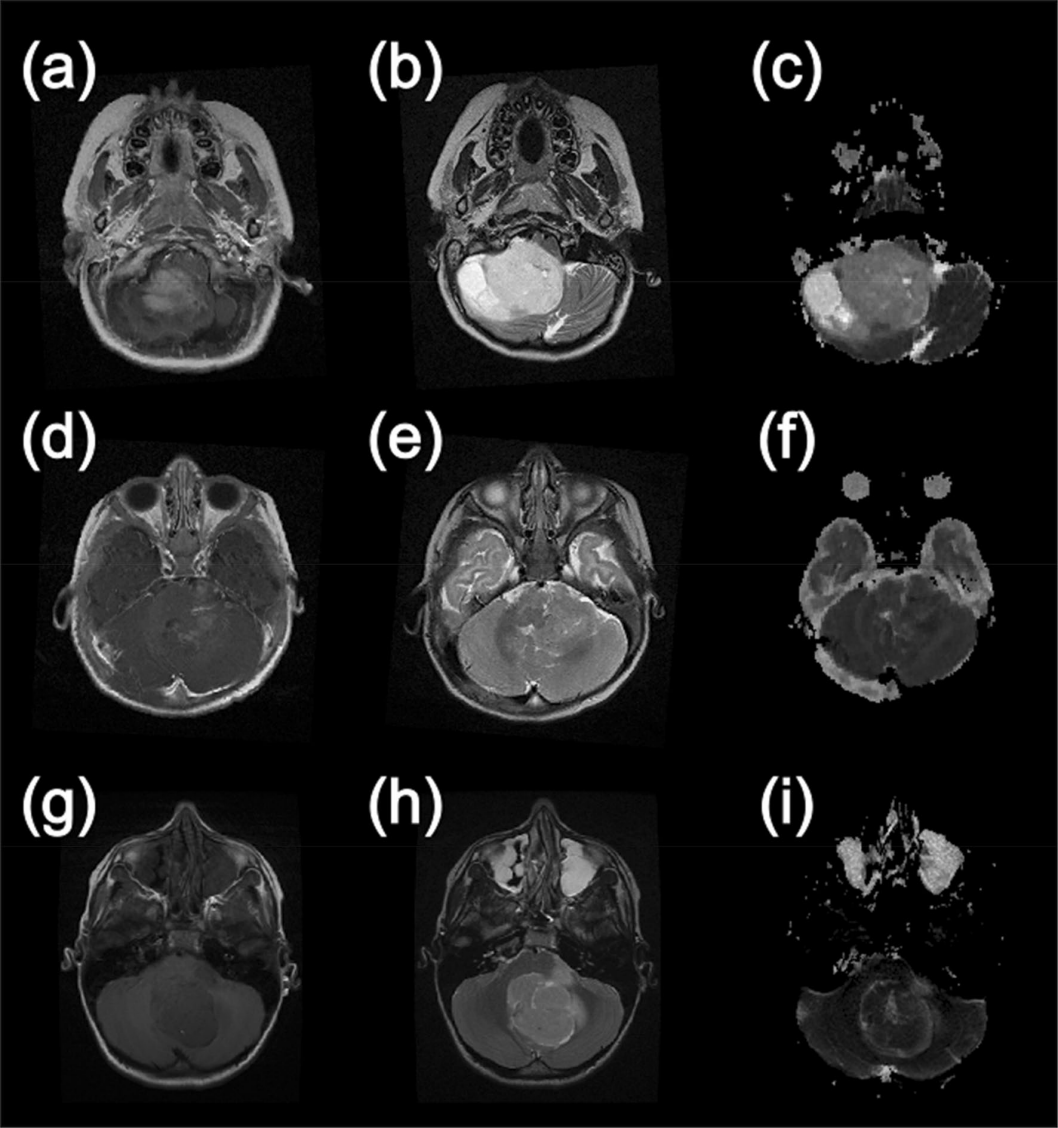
Example MR images from paediatric brain tumour patients. This first column shows T1-weighted images following the injection of gadolinium contrast agent. The second column shows T2-weighted images and the final column shows apparent diffusion coefficient maps calculated from diffusion-weighted images. (a–c) are taken from a patient with a Pilocytic Astrocytoma, (d–f) are from a patient with an Ependymoma and (g–i) were acquired from a patient with a Medulloblastoma.
